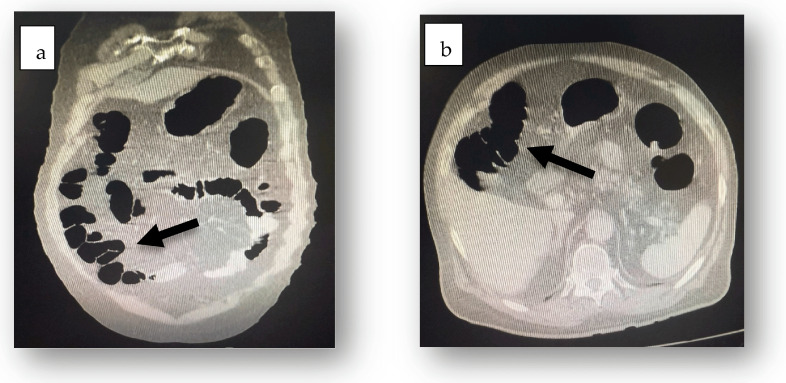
CT scan revealed intraluminal air in both the small bowel and colon (black arrow), consistent with aeroenteria (a) and aerocolia (b), respectively.
